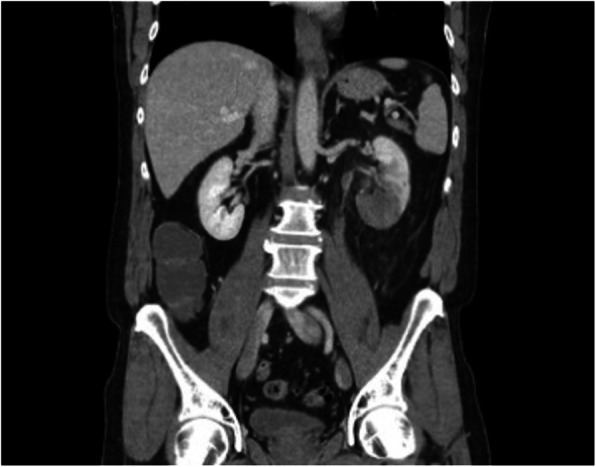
CT scan demonstrating absence of perfusion in the lower pole of the left kidney
